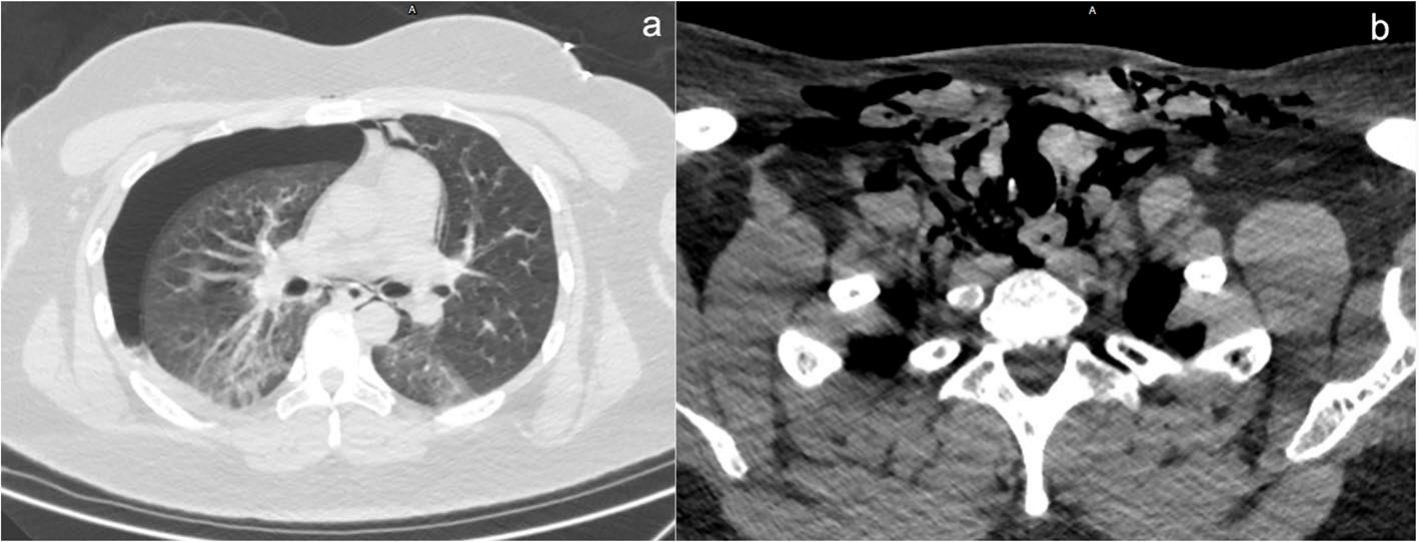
Chest and cervical computed tomography results. a Right pneumothorax; b Severe subcutaneous pneumatosis and partial rupture of the trachea at the level of thyroid isthmus
